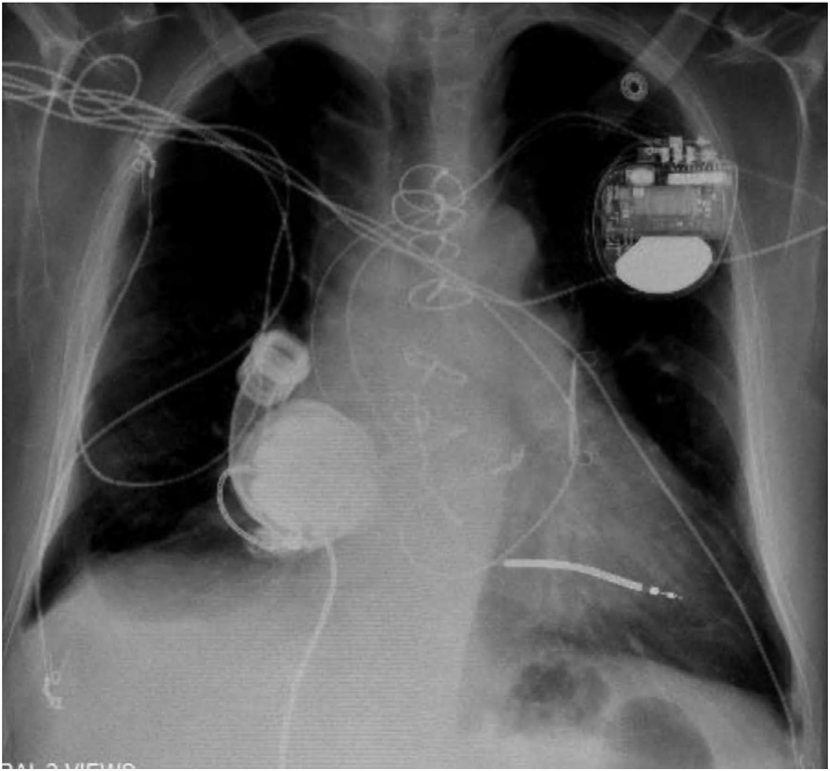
Chest radiograph demonstrates HeartMate 3 positioning in the right side of the chest.
